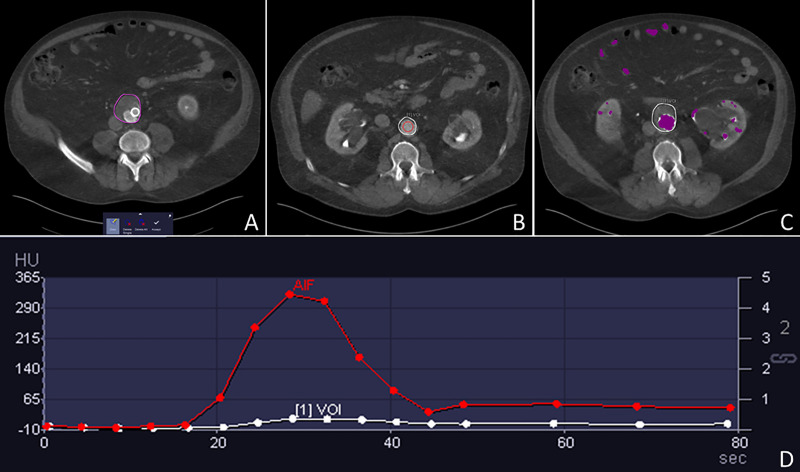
Post-processing steps involved using the CT Myocardial Perfusion software. 70-year-old man status post EVAR 3 years prior to the dCTA examination. After an initial manual segmentation step of the aorta (A), segmentation propagates automatically. A ROI is positioned in the aortic lumen (B) which is then automatically defined (C) in order to compute the AIF (D).
